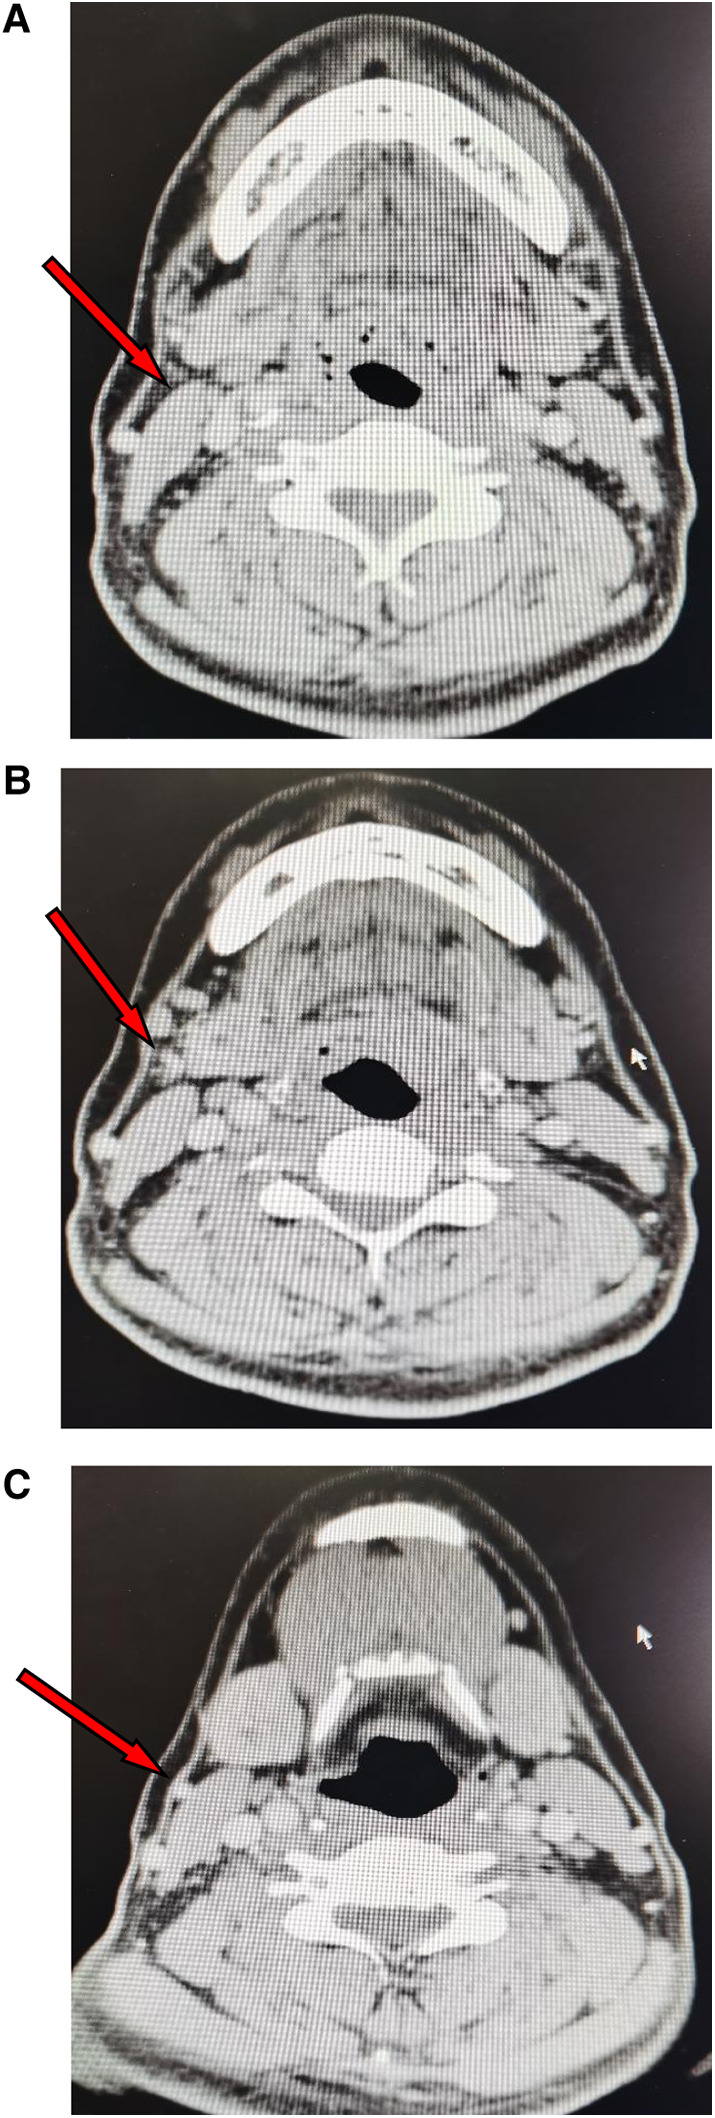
CT images showing the reflux vein of the submetal flap (arrows). (A) The reflux vein in the submandibular gland area. (B) The reflux vein drainaging into the external jugular vein. (C) The external jugular vein.
